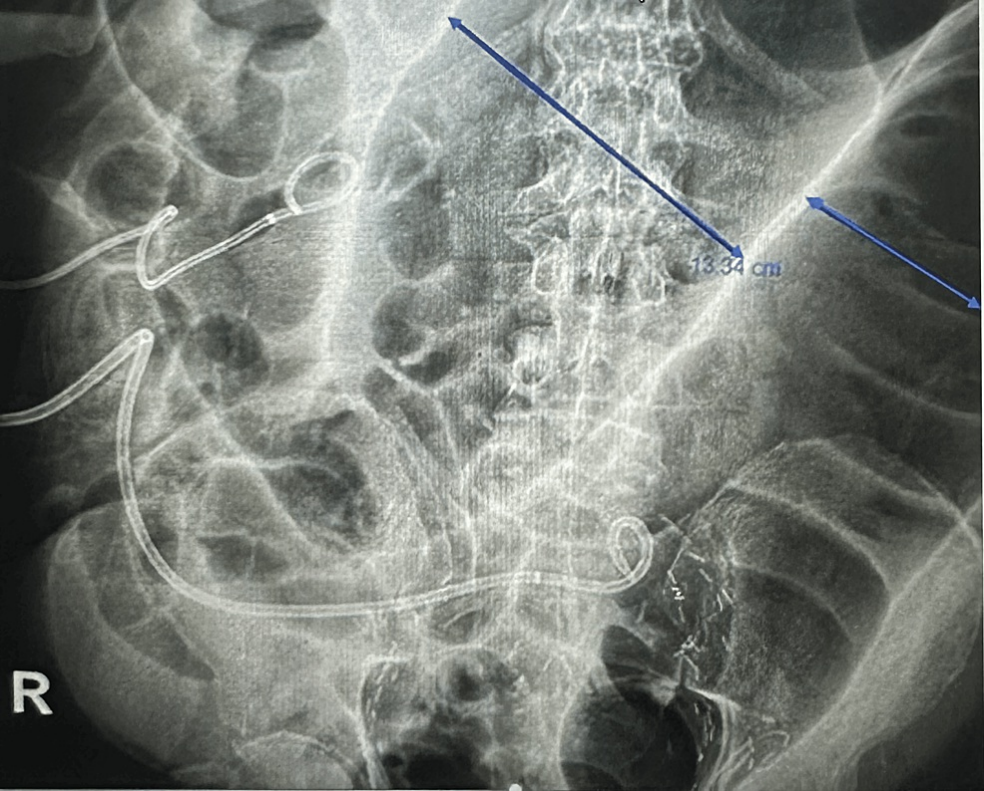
Abdominal X-ray showing extensive colonic distention up to 13.34 cm.
